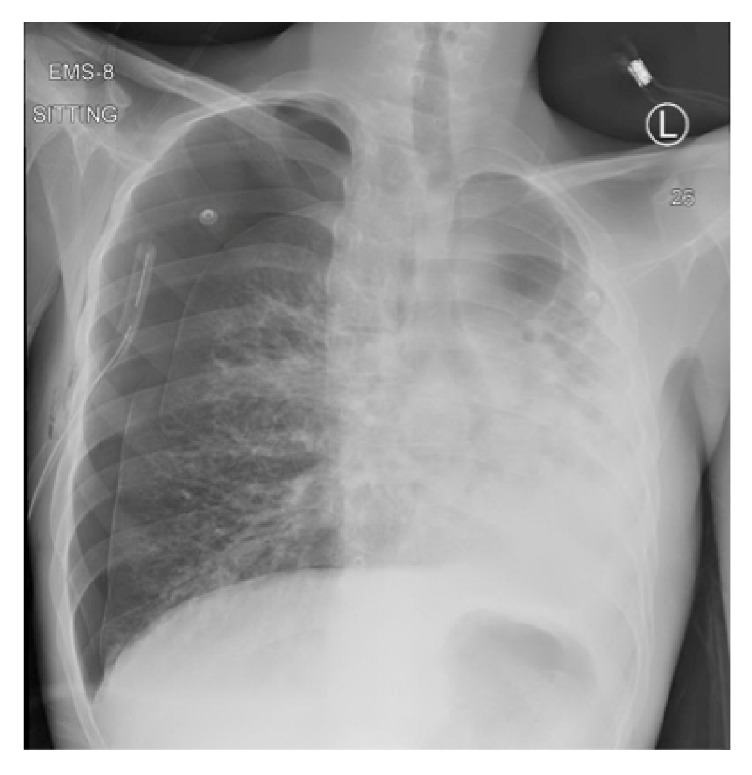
Chest X-ray after insertion of the chest tube showing improvement in the right-sided pneumothorax with subcutaneous emphysema.
